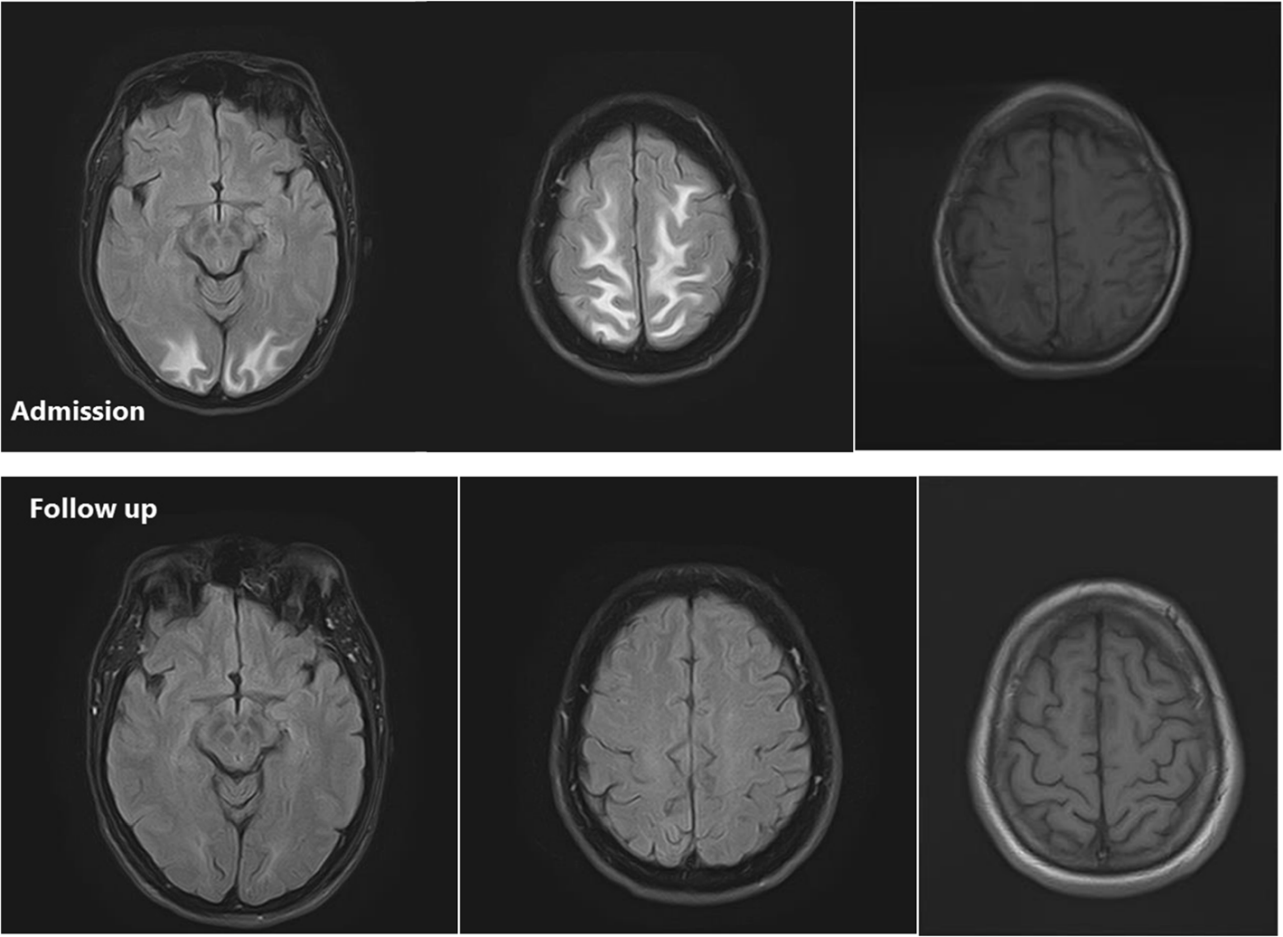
(Case 1): On admission MRI: MRI shows bilateral symmetric involvement of two areas in parasagittal parietal and occipital lobes. Gray matter, gray white matter junction, and the subcortical white matter are involved. The lesions are bright on T2-weighted and FLAIR images and hypointense on T1-weighted images. No evidence of diffusion restriction is noted. Follow-up MRI: Lesions completely resolved on the repeat MRI without chelation therapy
